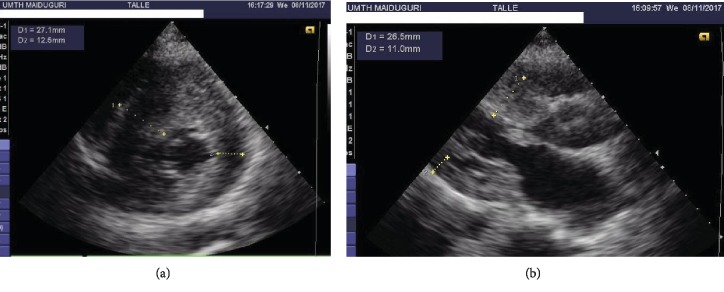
Parasternal short axis (a) and long axis (b) views showing asymmetric septal hypertrophy.
